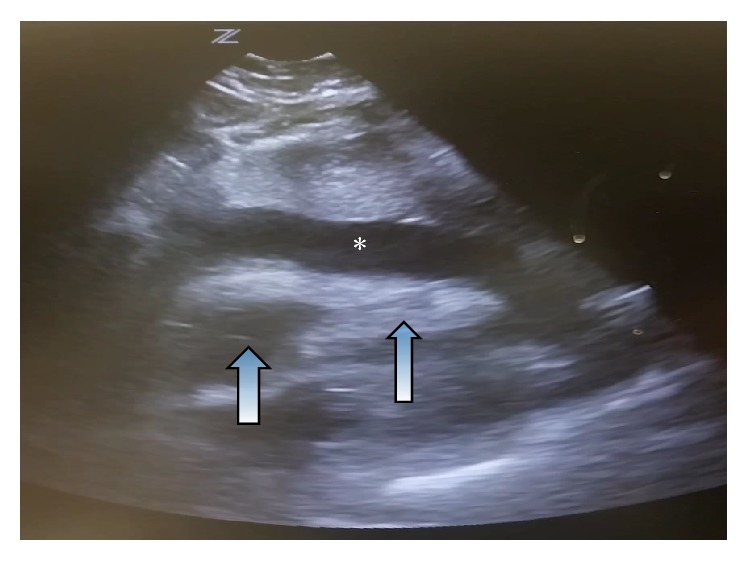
Subxiphoid window on bedside echocardiography demonstrating a large pericardial effusion (indicated by the *∗*) associated with right ventricular (RV) and right atrial (RA) collapse (indicated by the arrows).
